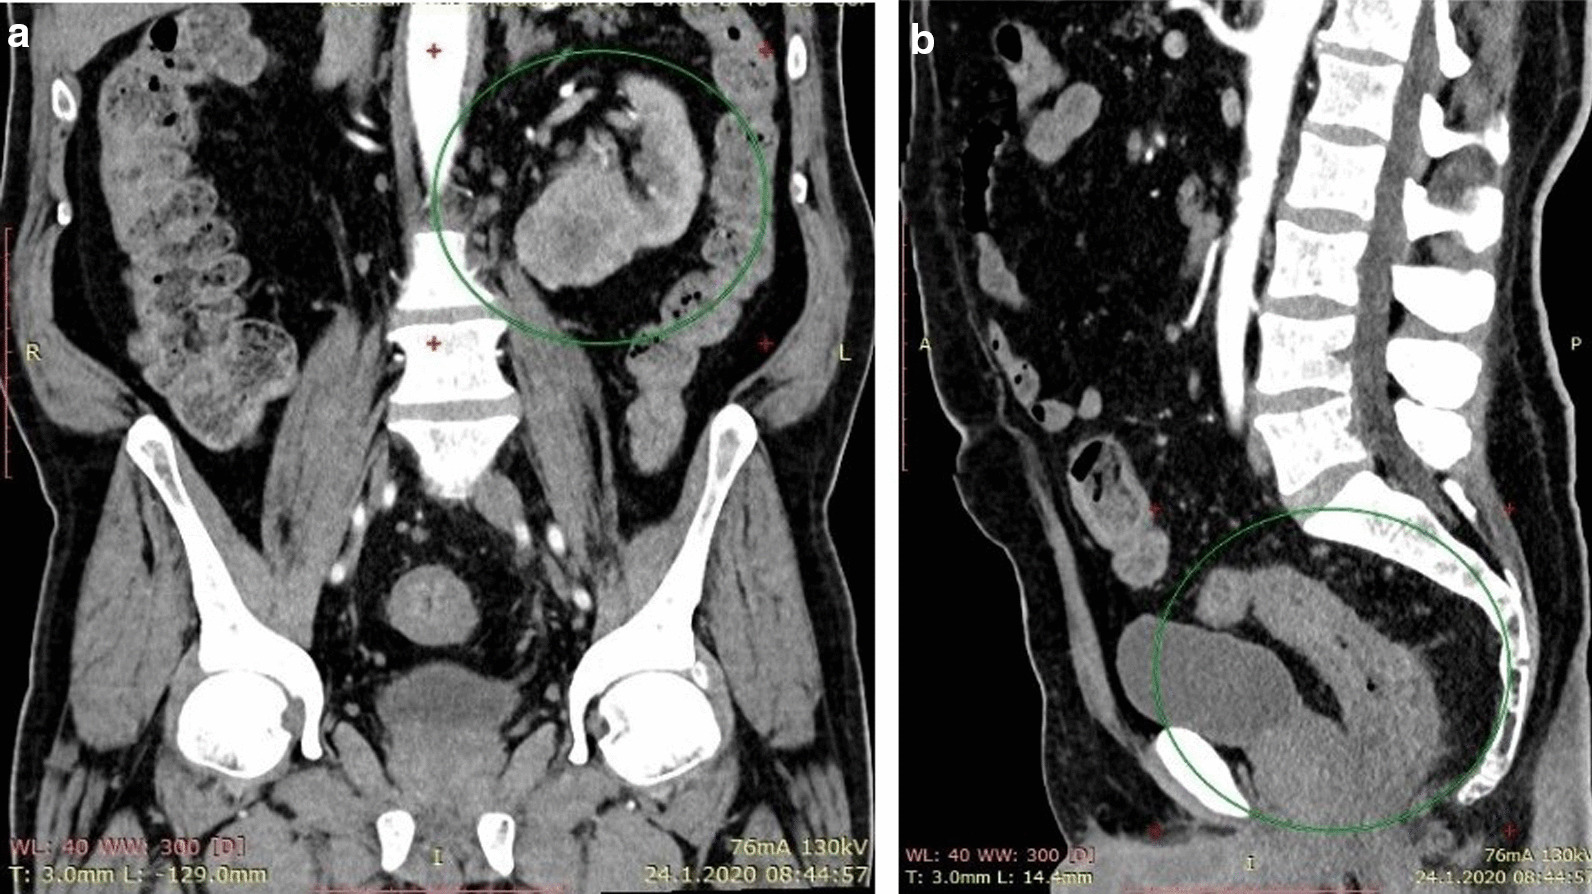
Computed tomography scan showing synchronous tumors (within green circles). a Kidney tumor in lower pole, b Rectal tumor mass with luminal narrowing
